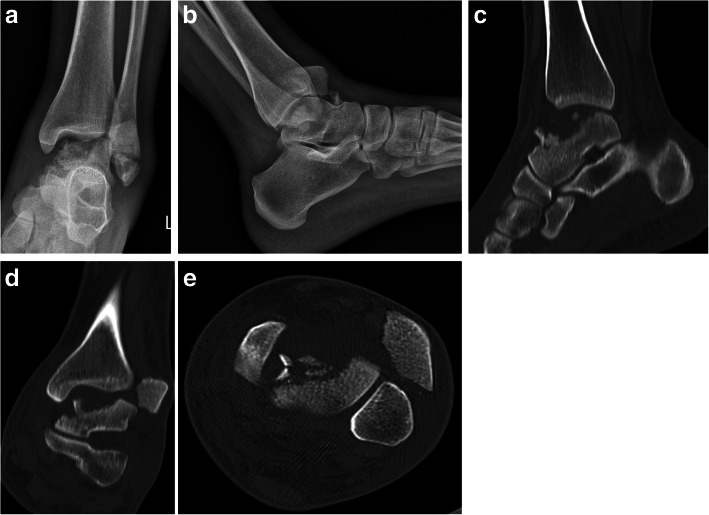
A big displaced partial talar body fracture in association with lateral malleolus fracture in a young woman. Radiographs (a & b), CT cuts (c-e)
